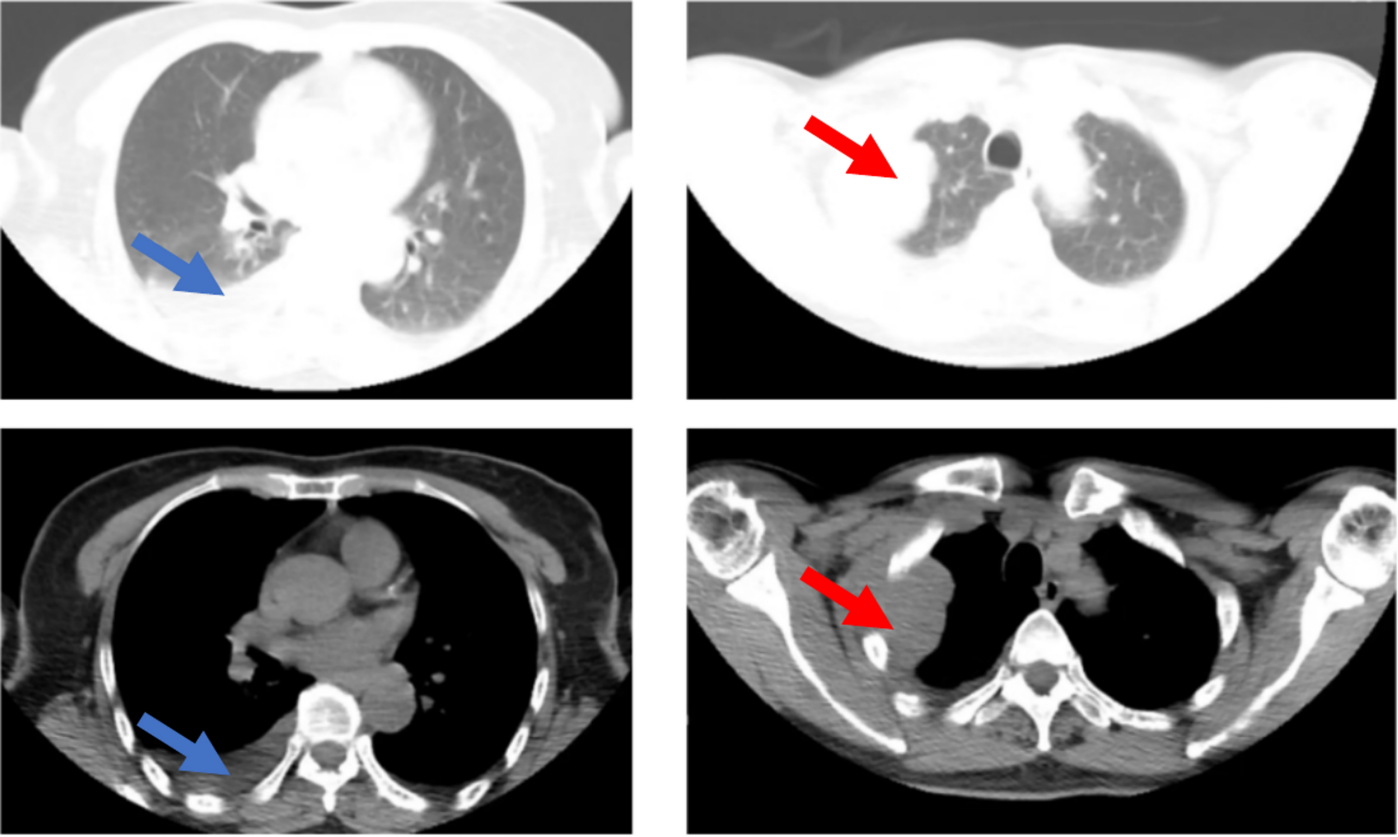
Non‐contrast chest CT scan of chest shows large right‐sided mass (red arrow) with osseous erosion of ribs 2–4 and intercostal muscle infiltration on May 5, 2024. This designation aligns with the imaging protocol chosen to avoid nephrotoxic contrast media in a patient with borderline low albumin (31.0 g/L). The well‐defined solid mass (oval shape with lobulated margins) is in the right upper lobe (segmental bronchus 3) with homogeneous attenuation (35–40 HU), mild pleural thickening adjacent to the mass and small right pleural effusions (blue arrow). CT, computed tomography.
